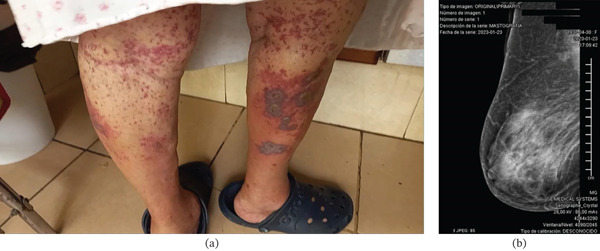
(a) Palpable purpura in lower extremities showing necrotic areas. (b) Mammography showed a high‐density irregular, partially spiculated mass with architectural distortion and fine, amorphous, segmental, and pleomorphic calcifications with diffuse skin thickening on the left breast corresponding with a BI‐RADS 5. Breast ultrasound reported BI‐RADS 4C (not shown).
